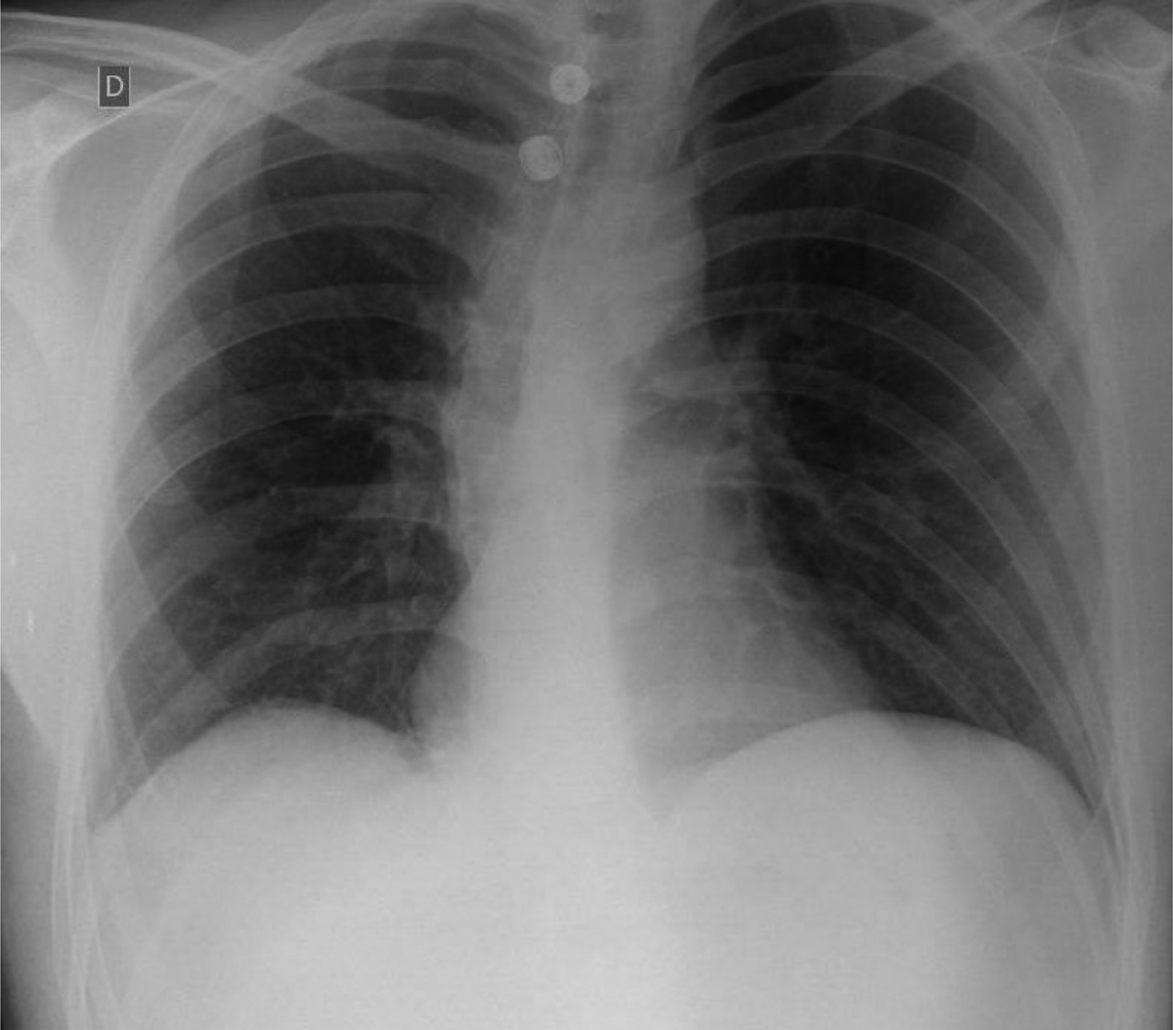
Postoperative X-ray 1 month after surgery.
